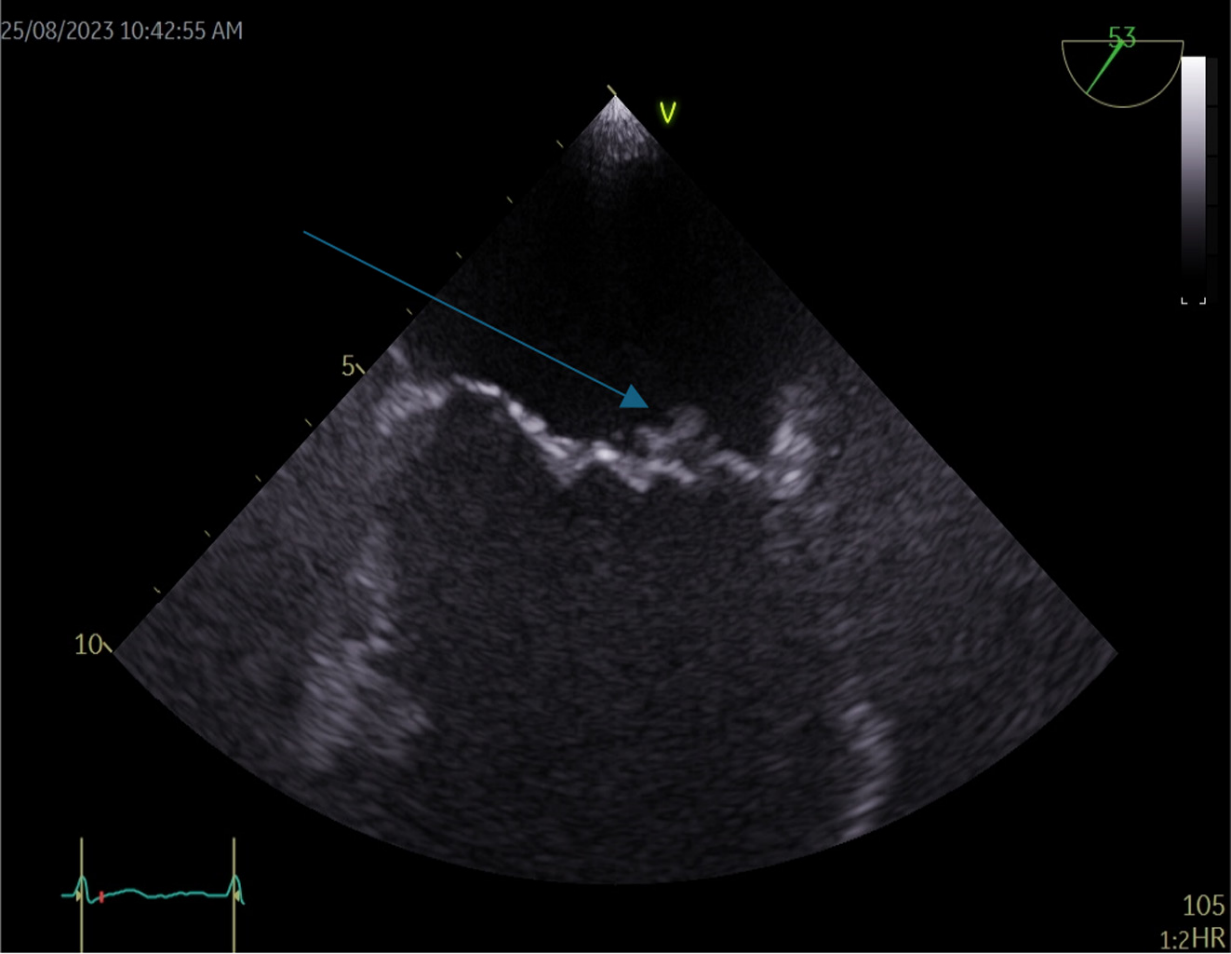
Longitudinal plane of the mitral valve from a transesophageal two‐chamber view delineating the left atrium in the upper part, the left ventricle in the lower part, and the mitral valve separating the two chambers: transesophageal echocardiography image suggestive of a vegetation in the posterior velum.
